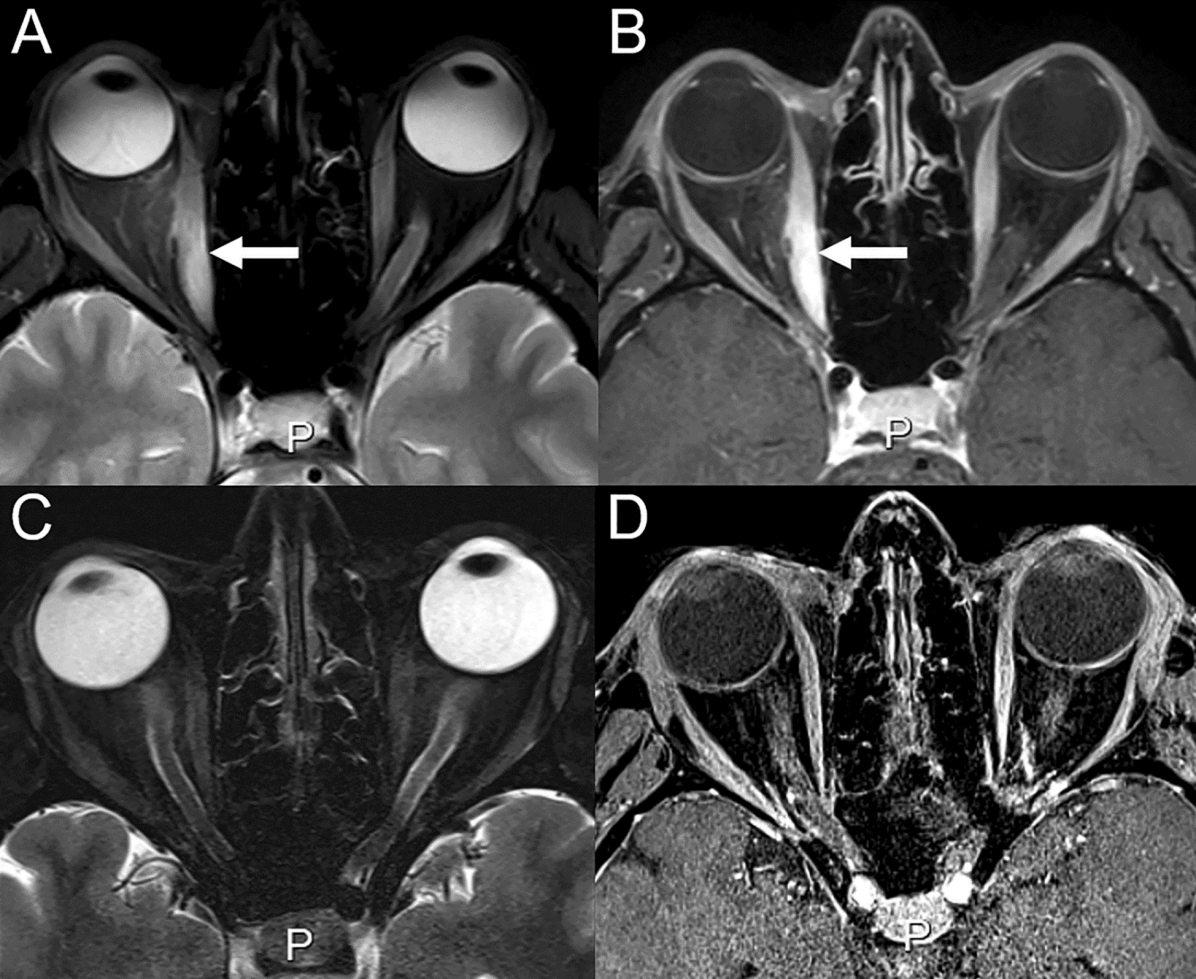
Immune-related orbital myositis in a 43-year-old female with cutaneous melanoma treated with ipilimumab (anti-CTLA-4) and nivolumab (anti-PD-1). After 3 cycles of ipilimumab and nivolumab, the patient reported diplopia. (A) On T2-weighted and (B) contrast-enhanced images, orbital edema, abnormal enhancement and thickening of the right medial occulomotor muscle can be seen, consistent with orbital myositis (arrows). (C, D) MRI at 1 month from treatment discontinuation with disappearance of signs of inflammation.
